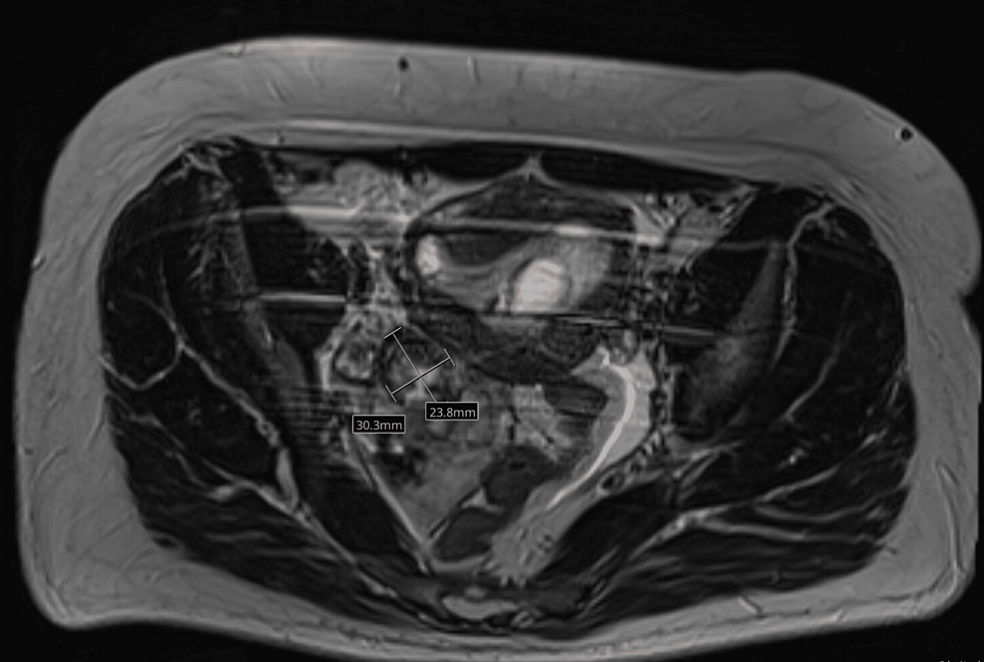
Cross-sectional MRI demonstrating a complex right adnexal lesion suggestive of an ectopic pregnancy with surrounding hemorrhage.
